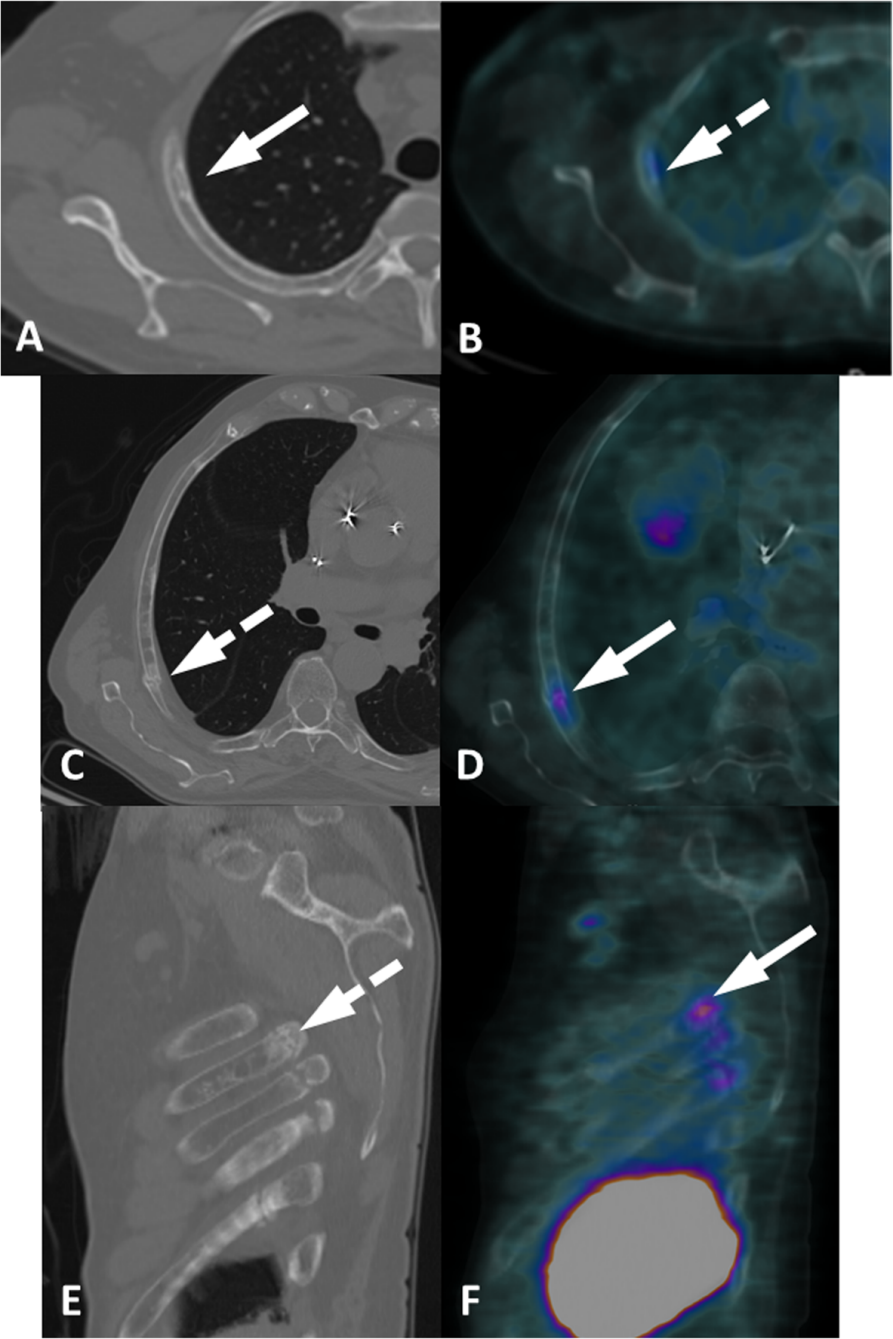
Focal PSMA uptake in the ribs in two different patients. Patient 1, fibrous osseus defect (a, b). Axial CT (a) and axial ^68^Ga-PSMA-PET/CT (b) show a single focus of faint uptake (dashed arrow) in a typical fibrous osseous defect of the third right rib (arrow). Patient 2, ribs fractures (c-f). Axial CT (c), sagittal CT (e), axial ^68^Ga-PSMA-PET/CT (d) and sagittal ^68^Ga-PSMA-PET/CT (f) show mild uptake (arrows) in continuous ribs fractures (dashed arrows)
